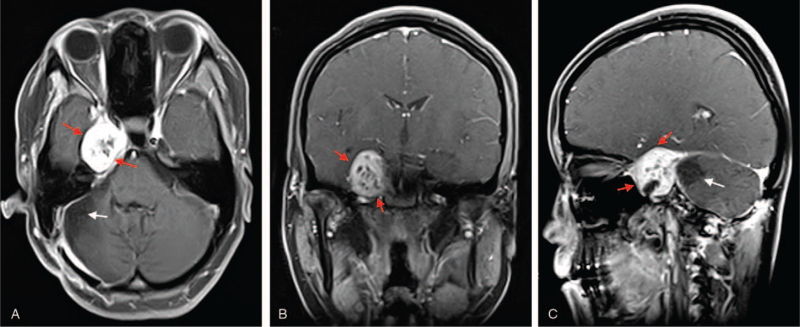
(A–C): Are first postoperative MRI showing tumor in the petrous part but no tumor in the posterior part. A = axial view, B = coronal view, C = sagittal view. *Red arrow = tumor, White = No tumor*.
